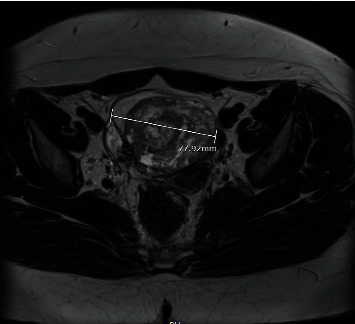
MRI findings. Right adnexal mass with a solid and cystic appearance.
